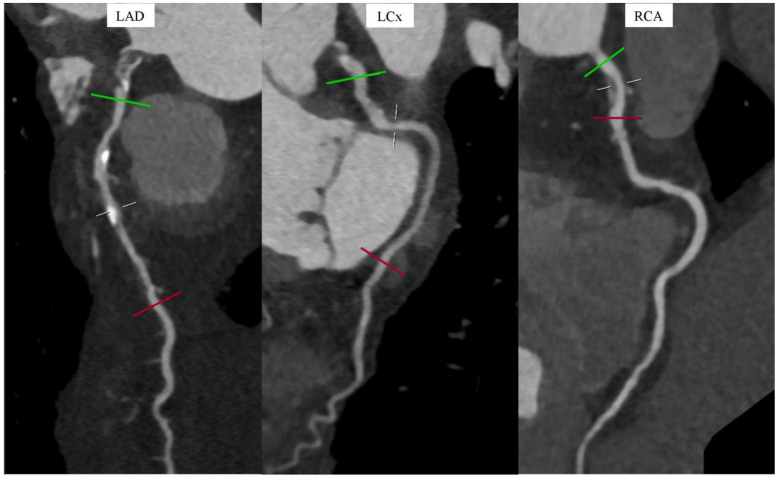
Coronary computed tomography angiography. Angiographic phase. Curved multiplanar reconstructions (cMPR) of the left anterior descending (LAD), the left circumflex (LCx), and the right coronary artery (RCA), (successive panels from the left). The green gate (solid green line) indicates the reference level of the nonstenotic arterial lumen above the level of stenosis. The red gate (solid red line) indicates such a reference level below the stenosis level. The white gate (dashed white line) indicates the level of maximum visualized coronary artery stenoses.
